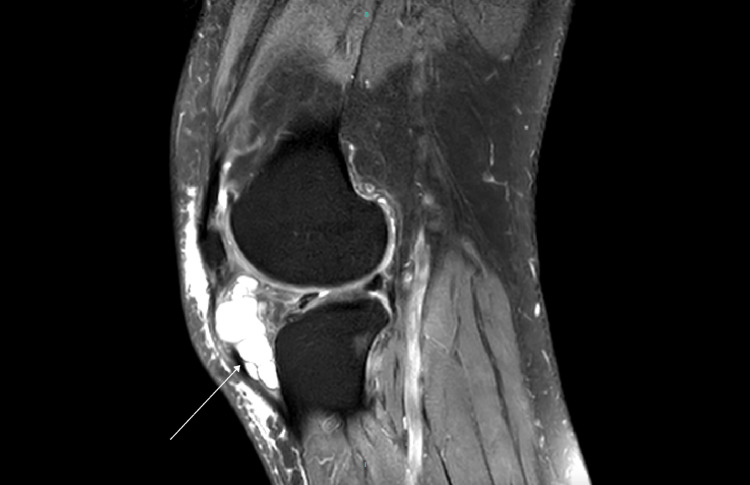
MRI demonstrating sagital view of the left knee with massive cyst extending from Hoffa's fat pad (white arrow).
